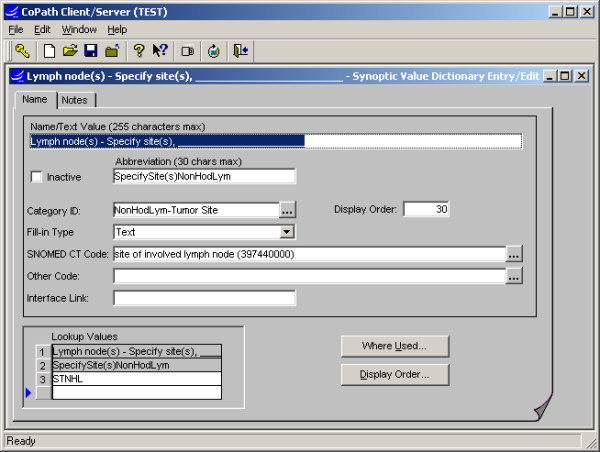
Synoptic Value dictionary interface: The Values to be applied to worksheets are defined or edited and attributes configured, e.g. to allow numeric or text fill-ins or link SNOMED CT code.
